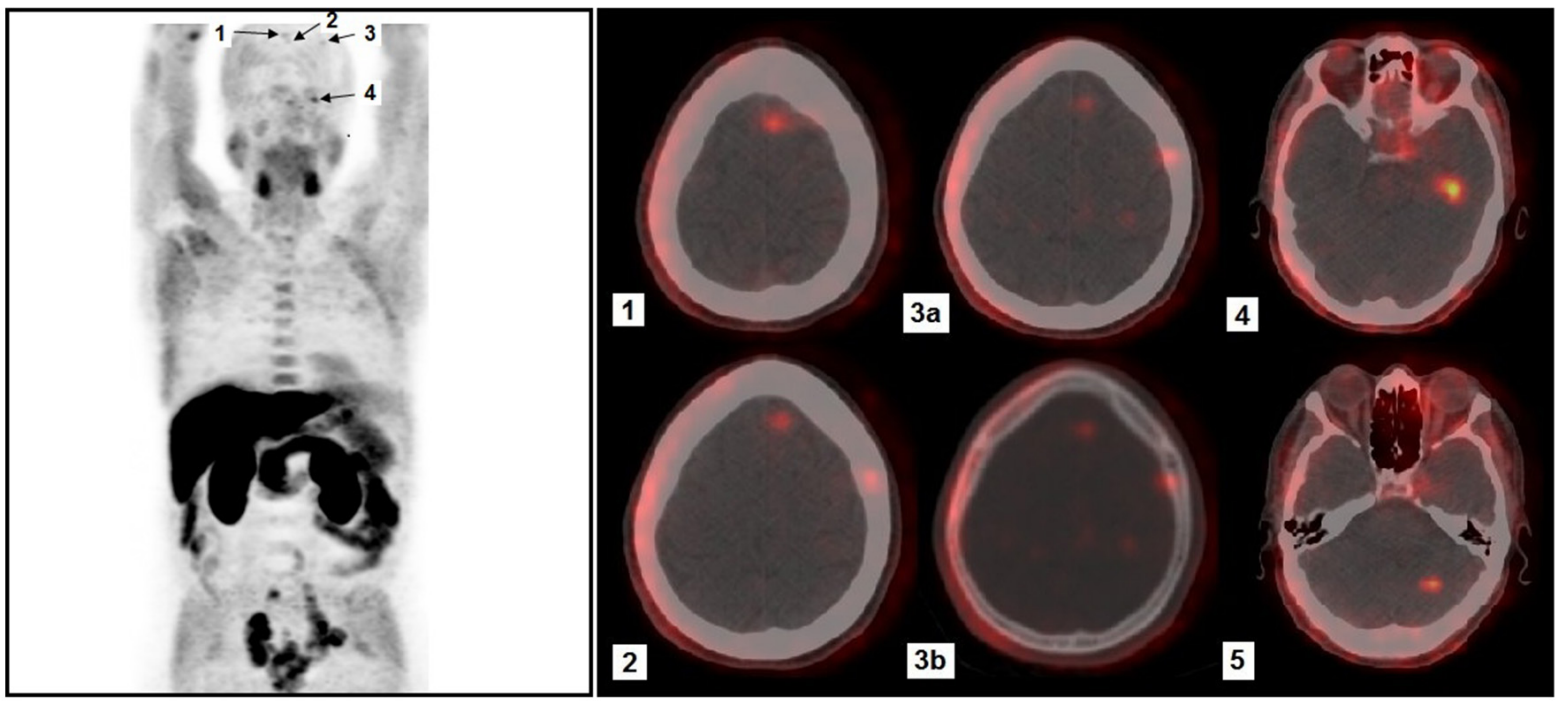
Whole-body maximum intensity projection (MIP) [18F]fluorocholine PET image (left) showing extensive pelvic lymph node involvement, as well as several intracranial foci (arrows). Physiologic tracer distribution is observed in the salivary glands, liver, pancreas and bowel. Axial fused PET/CT images (right) show intracranial foci: (1, 2) Two small paramedian foci located in the left frontal lobe at different axial levels, with very faint uptake. (3) Small focus located in the left lateral frontal lobe, with very low-level uptake; although it appears close to the skull on fused images, CT in bone window (3b) confirms the absence of skull involvement, indicating an intra-parenchymal lesion (3a). (4) Focus located in the left temporal lobe, demonstrating more conspicuous uptake. (5) Focus located in the left cerebellar hemisphere.
